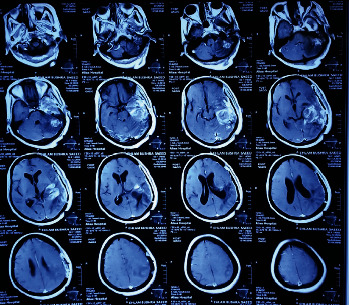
MRI brain axial cut T1-weighted image with contrast shows left temporal heterogeneously enhancing lesion featuring malignancy.
